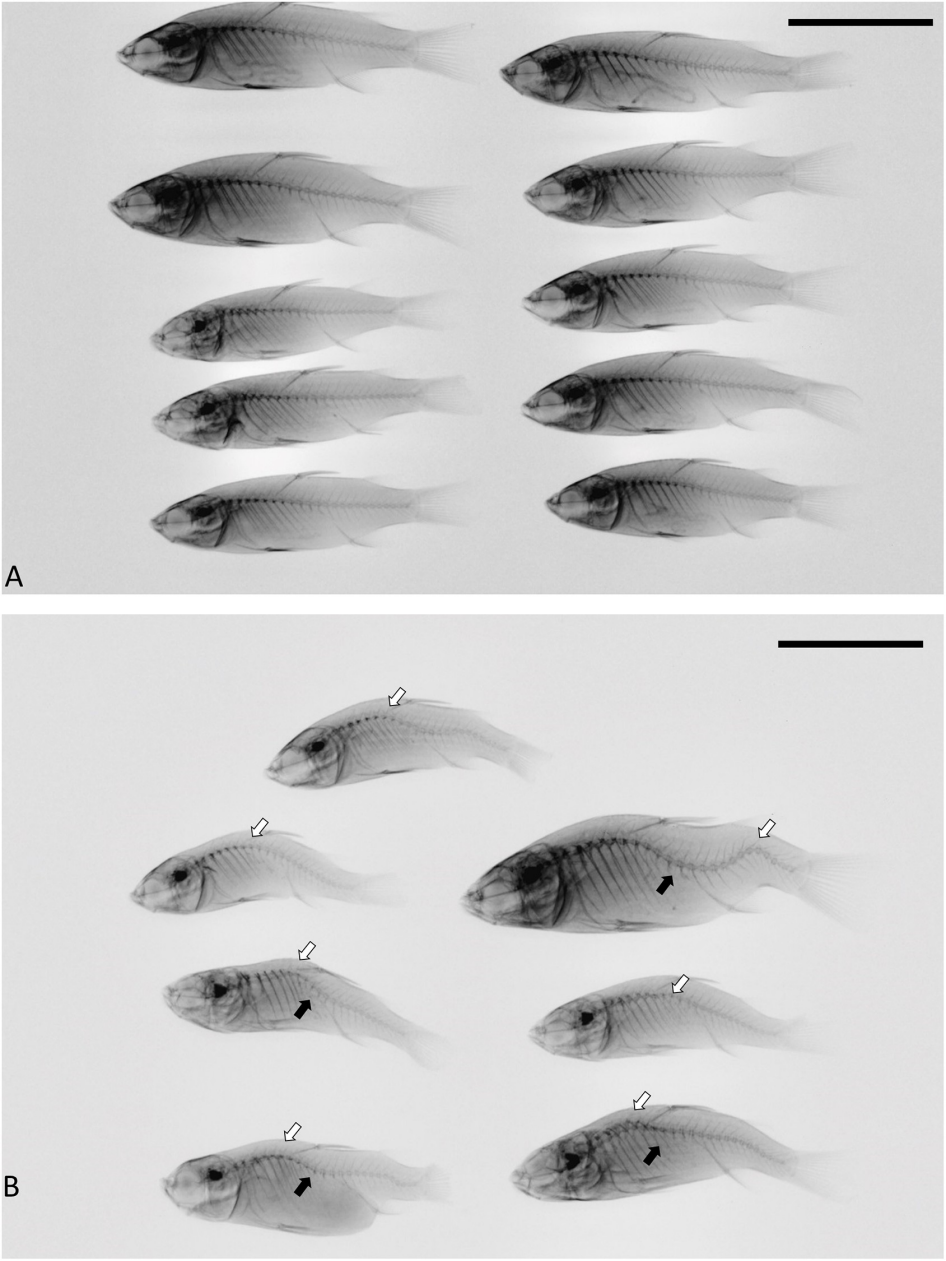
Lateral X-ray views of largescale mullet in Experiment 2. (A) Fish were transferred to 26°C after the heat-treatment stage, or (B) exposure to 36°C was continued for another 8 weeks. Open arrows indicate kyphosis, and solid arrows indicate lordosis. Scale bar, 10 mm.
